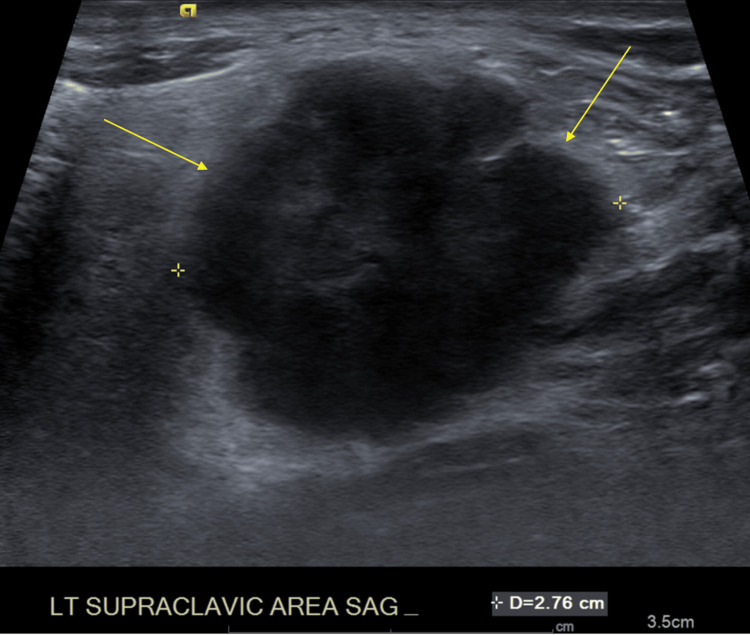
Ultrasound of the left supraclavicular region, highlighting lymphadenopathy marked by yellow arrows.
